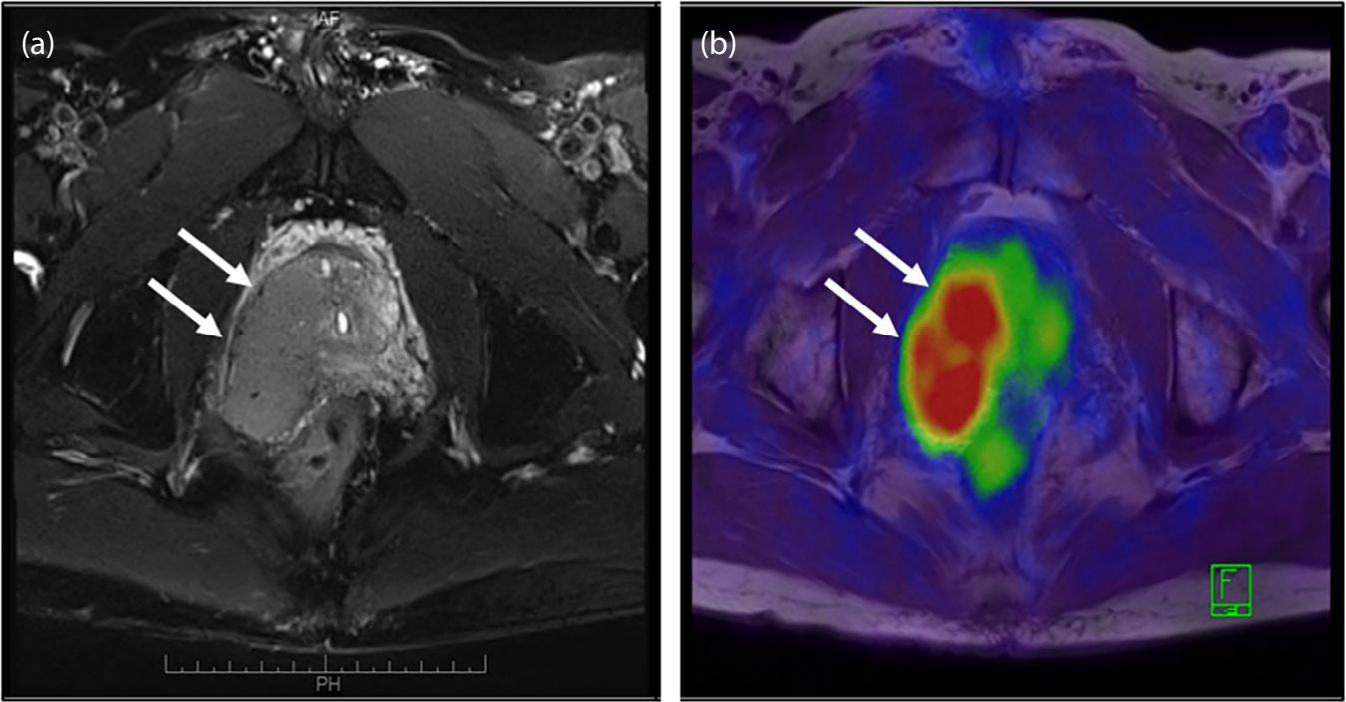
Preoperative fluorodeoxyglucose‐positron emission tomography/MRI. (a) T2‐weighted low‐signal lesion in the right lobe. (b) FDG accumulation with SUV max 7.1.
